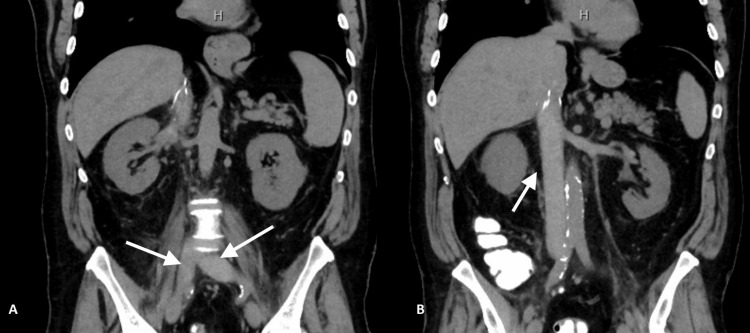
CT scan of the abdomen and pelvis CT scan coronal section showing evidence of fullness of bilateral common iliac veins (arrows in A) and the inferior vena cava (arrow in B) suggestive of extensive thrombosis.
